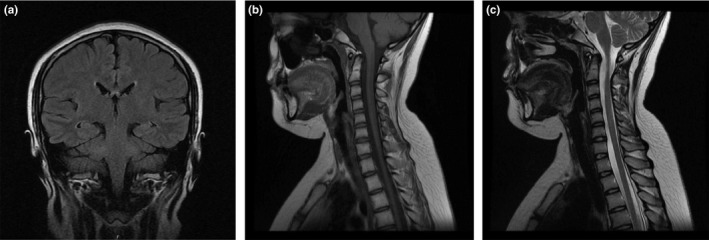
MRI‐scan after treatment. Coronal brain MRI FLAIR image showing no significant change in the appearance of the previous lesions. Cervical cord MRI image showing no significant change in the appearance of the prior lesions, and the appearance of the spinal cord was normal. (a) Coronal brain T2‐FLAIR. (b) Sagittal cervical cord T1. (c) Sagittal cervical cord T2
